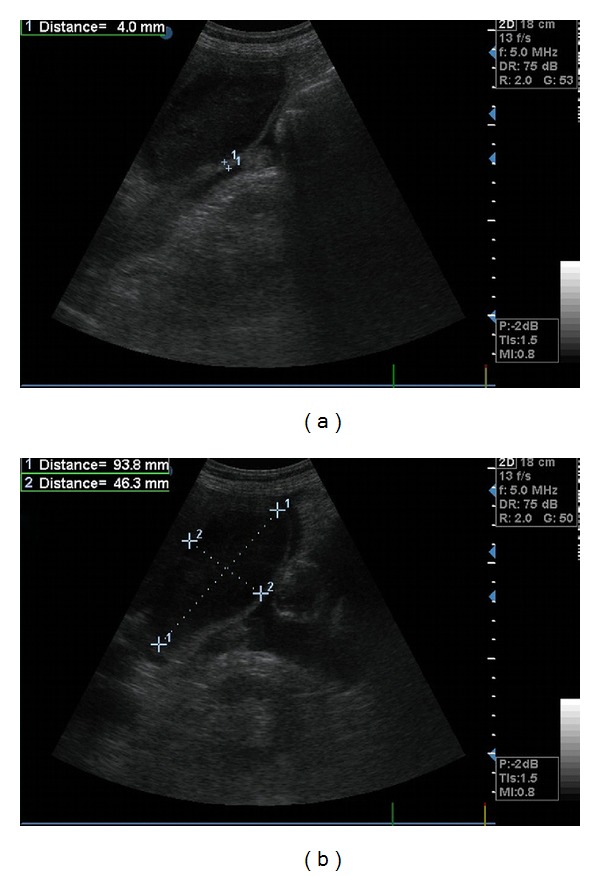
Gallbladder ultrasound depicting one patient with acute acalculous cholecystitis exhibiting wall thickening (4 mm) in the presence of sludge (a) and marginally increased dimensions (93 × 46.3 mm) with pericholecystic fluid (b).
